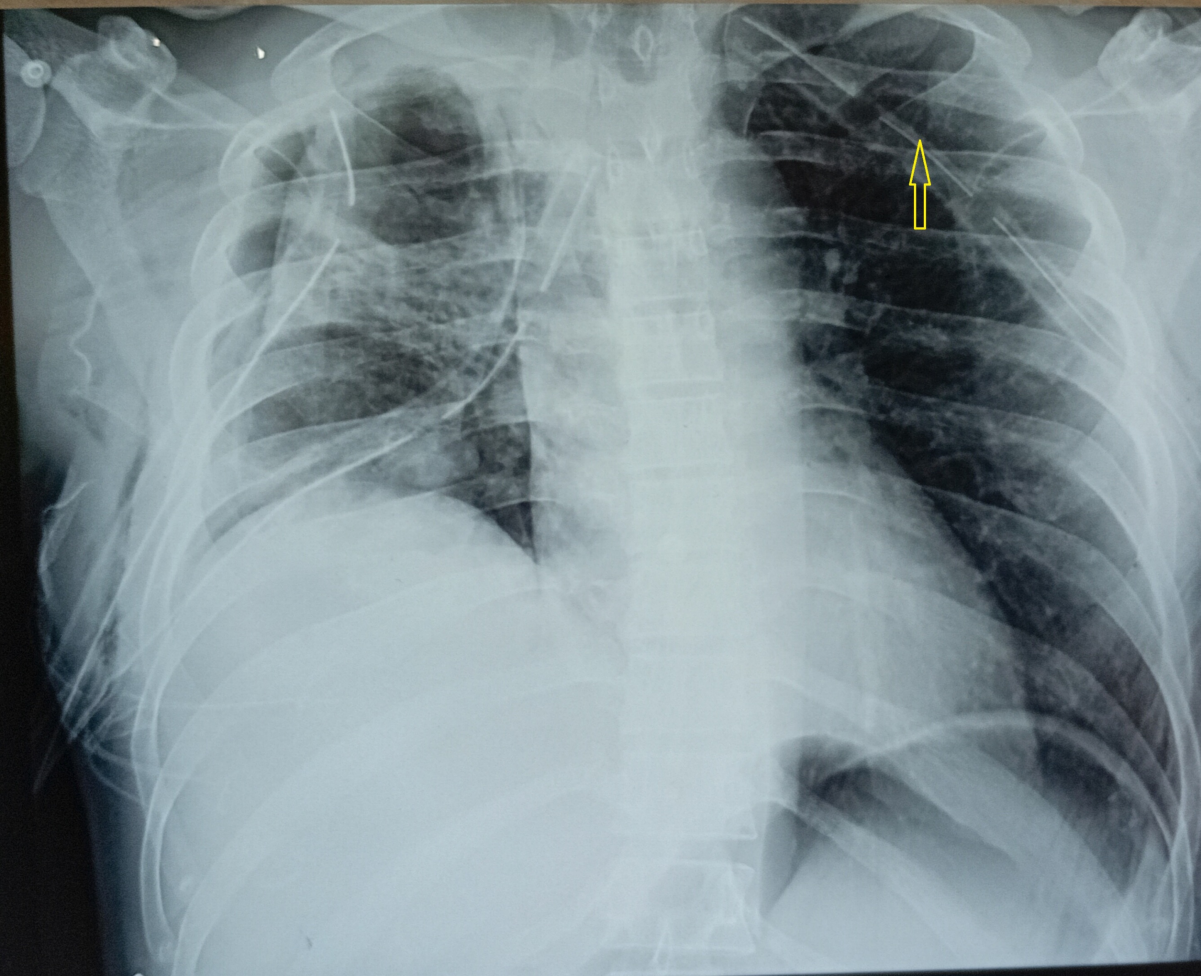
Chest X-ray showing multiple intercostal tubes; left sided tube adjacent to the mediastinum (yellow arrow).
